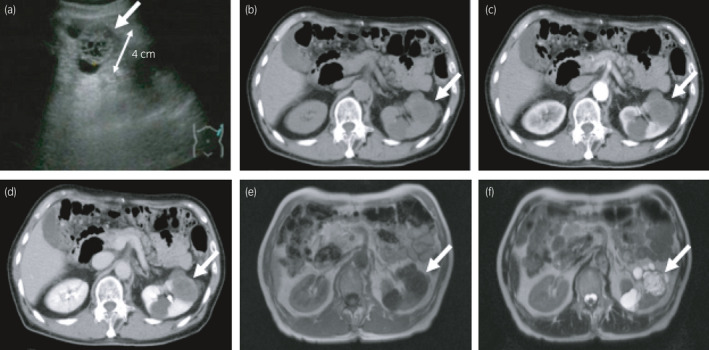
Images of the multilocular cystic tumor with septa (white arrows) at the central pole of the left kidney. (a) An image of ultrasonography, (b) plain CT, (c) contrast‐enhanced CT in an early phase, and (d) contrast‐enhanced CT in a late phase. Septa located in the tumor showed a contrast effect. Images of MRI are displayed in panels e and f. (e) T1‐weighted image (f) T2‐weighed image. The multilocular cystic tumor showed low intensity on the T1‐weighted image and high intensity on the T2‐weighted image.
